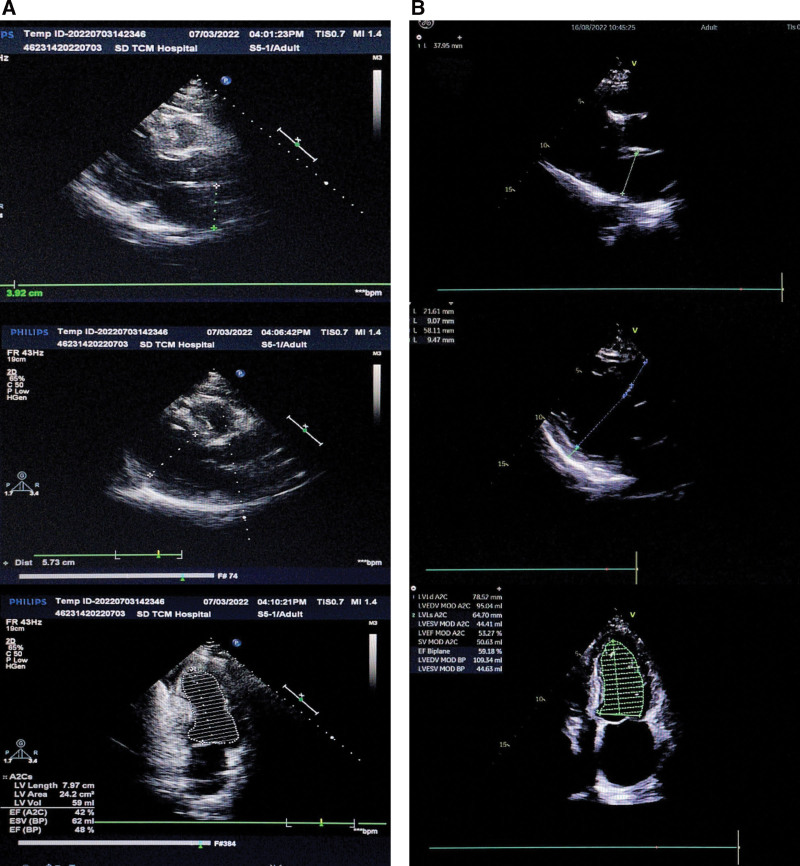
(A) Cardiac ultrasound within 2 mo outside the hospital. (a) Cardiac ultrasound on July 03, 2022: myocardial lesions; left ventricle slightly larger LAED 39 mm, LVED 57 mm); mitral regurgitation (mild); tricuspid regurgitation (mild); and reduced left ventricular systolic function (LVEF 0.48). (B) Cardiac ultrasound on August 16, 2022: left ventricle slightly larger (LAED 38 mm, LVED 58 mm); mitral regurgitation (mild); tricuspid regurgitation (moderate); and pulmonary hypertension; (LVEF 0.59). LAED = left atrium end diastolic, LVED = left ventricular end diastolic diameter, LVEF = left ventricular ejection fraction.
